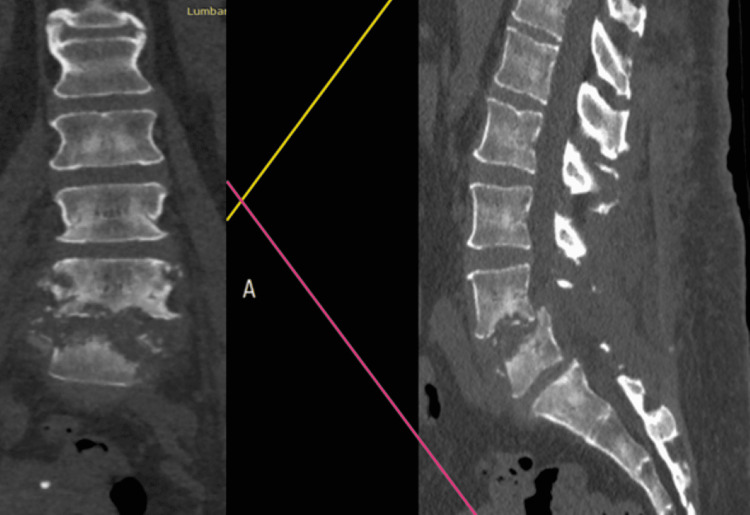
Preoperative of the first surgical stage, from left to right: coronal and sagittal CT slides. T1 showing a destructive process both (L4-L5) endplates and vertebral bodies destruction, starting from the ventral portions of both structures, anterolisthesis of L4 is also observed with stenosis L4-L5.
